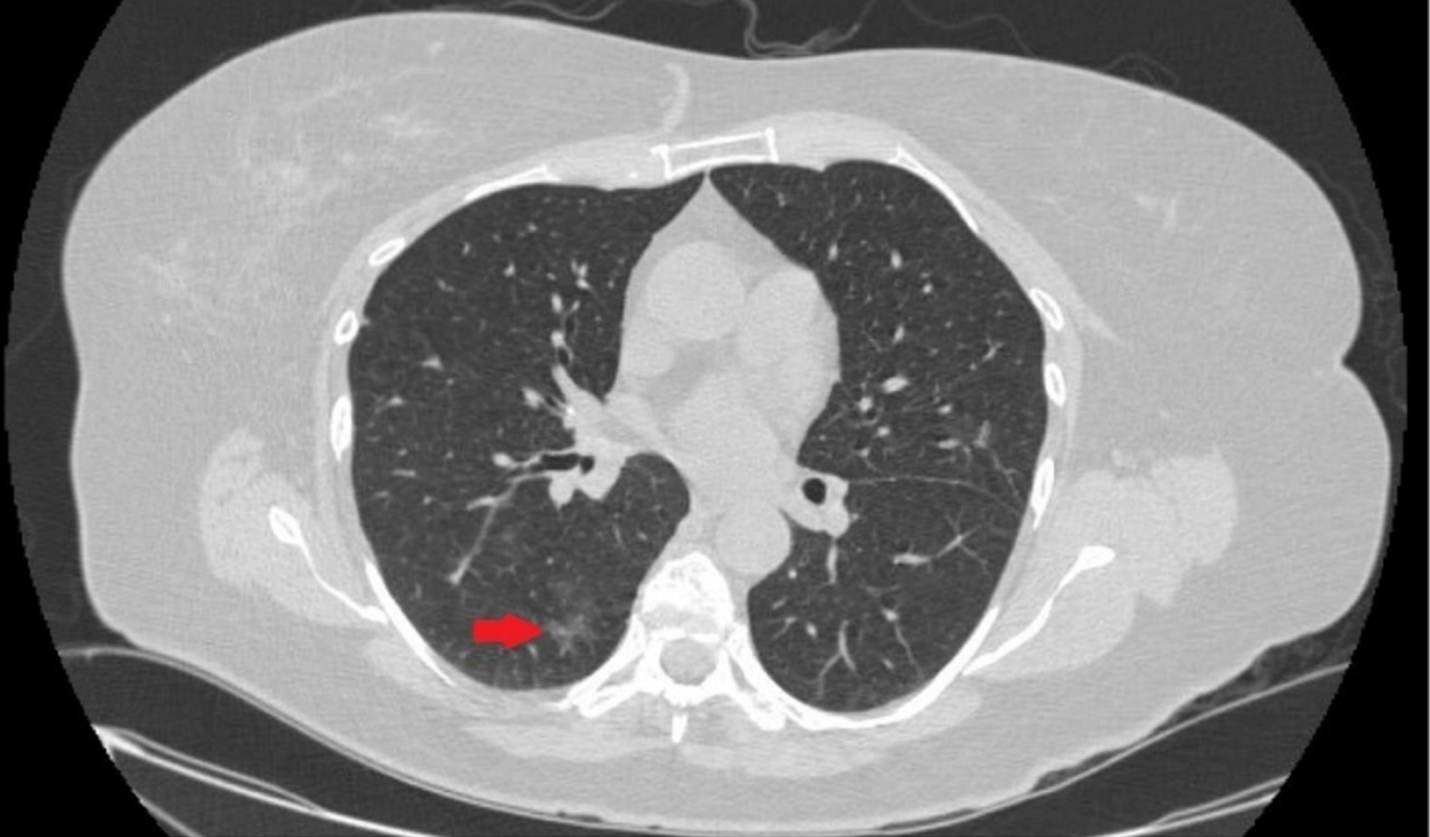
Computed tomography (CT) scan of the thorax showing ground glass infiltrate in the right lower lobe (red arrow)
